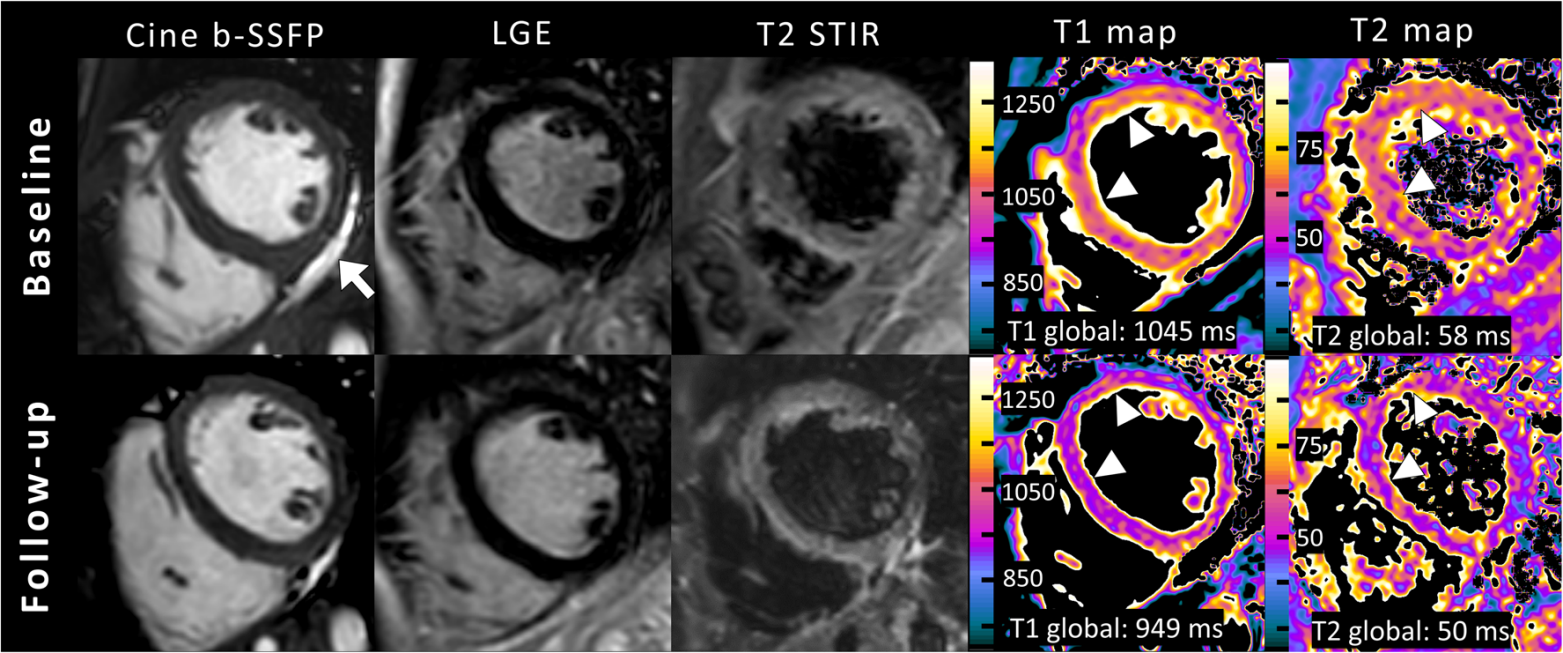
A clinical example of cardiac magnetic resonance imaging (MRI) in short-axis view in a 16-year-old boy. Cine images (balanced steady state free precession [b-SSFP]) show normal left ventricular ejection fraction (LVEF; 58%, no segmental hypokinesia) and pericardial effusion basal inferior (*arrow*). No focal or diffuse enhancement was identified on late gadolinium enhancement (LGE). No focal myocardial edema was visible on fat-suppressed (T2-weighted short TI inversion recovery [T2 STIR]) images. Mapping parameters displayed high global myocardial native T1 and T2 relaxation times at baseline cardiac MRI and normalization at follow-up (*arrowheads* show the most affected segments). The diagnosis of acute diffuse myocarditis in this patient was only possible using quantitative parameters according to 2018 Lake Louise criteria and would have been missed by the original Lake Louise criteria
